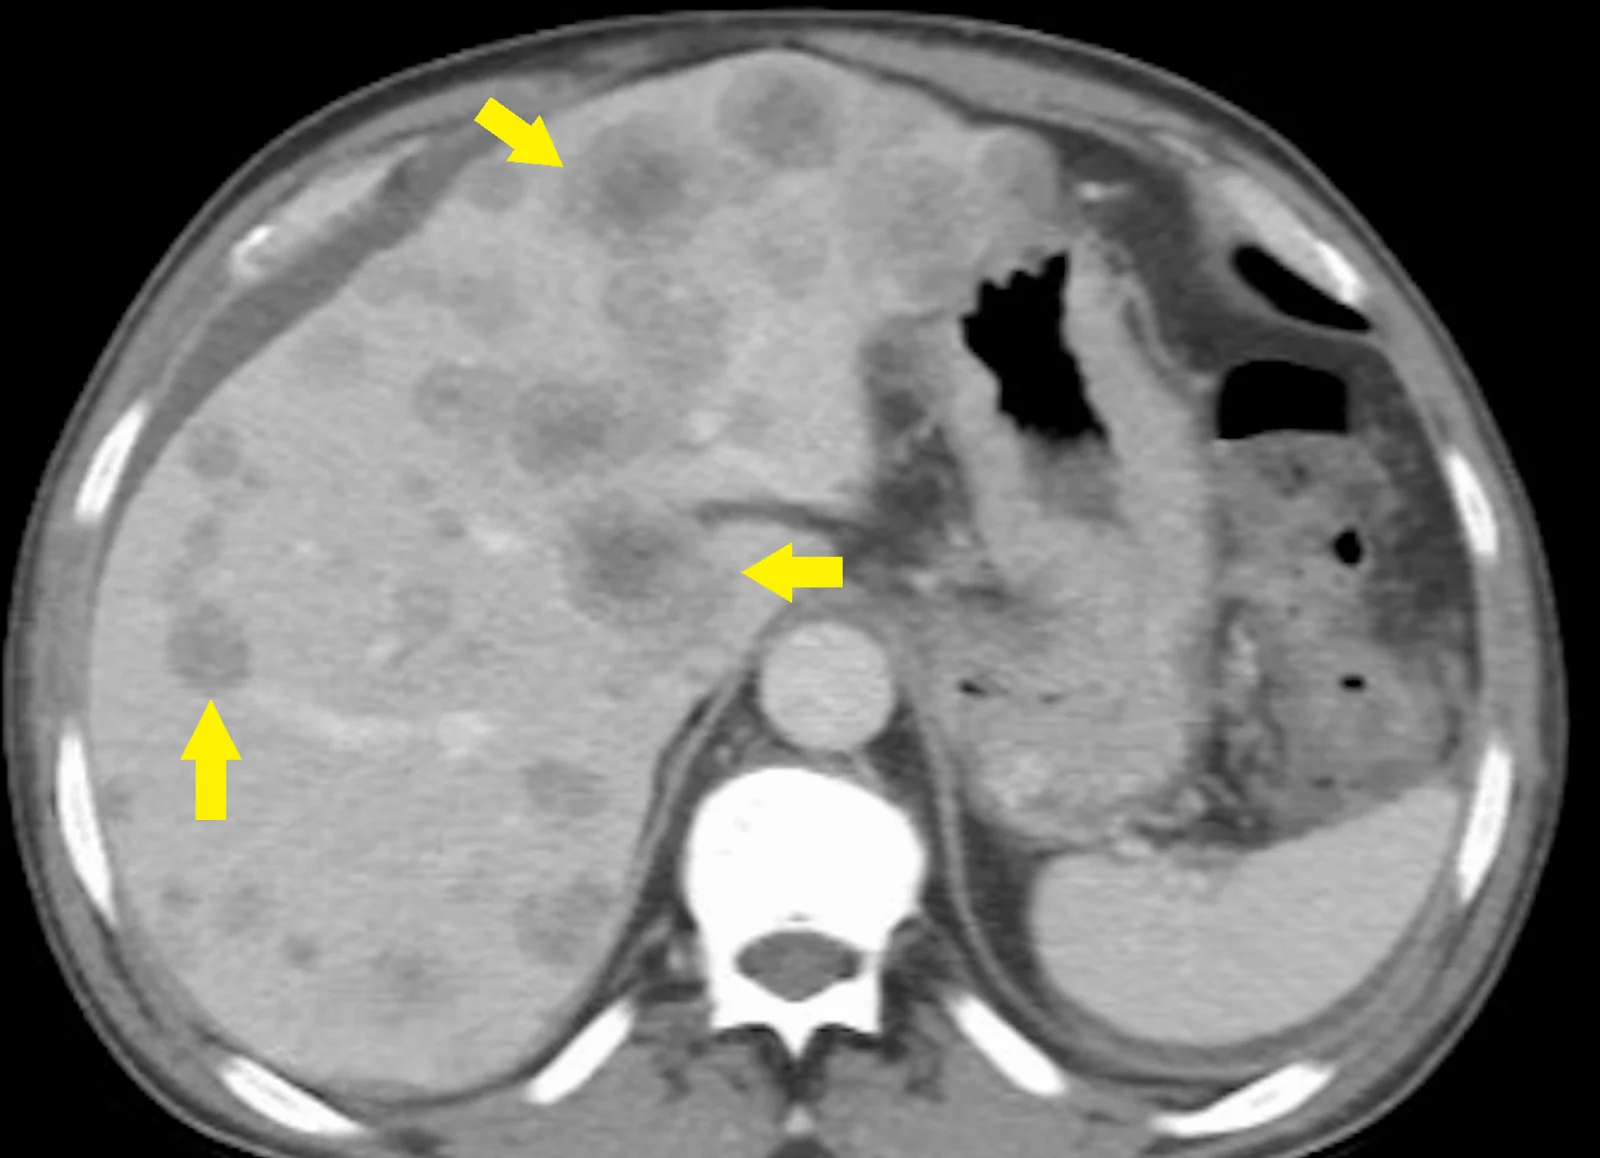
CT scan of the abdomen demonstrating numerous hepatic metastases The yellow arrows indicate representative hypodense hepatic lesions consistent with metastases.
